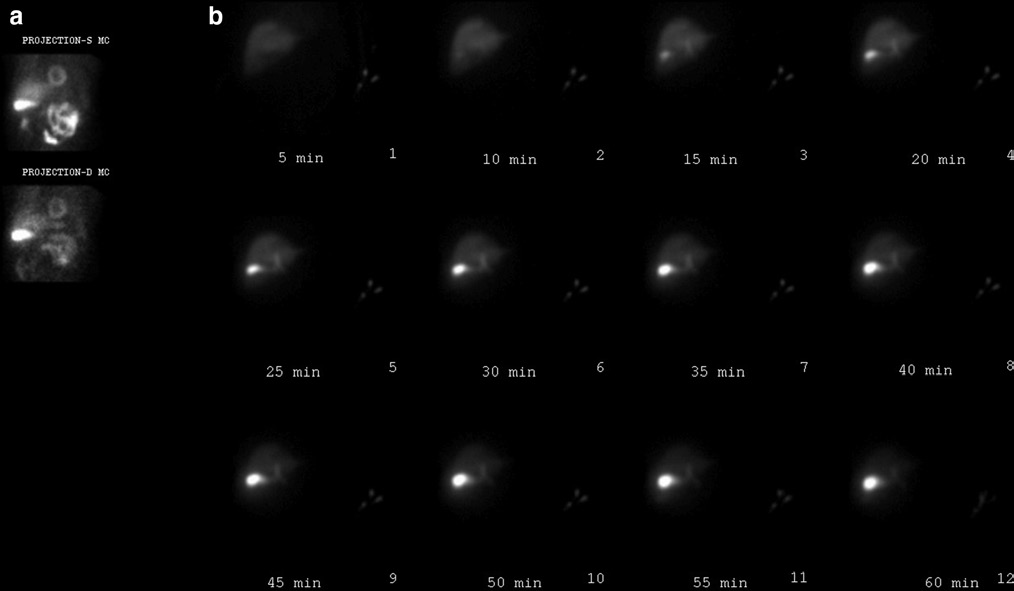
GB visualized on the stress/rest MIBI (a) and HBS data (b). GB, gall bladder; HBS, hepatobiliary scintigraphy; MIBI, methoxy isobutyl isonitrile.
